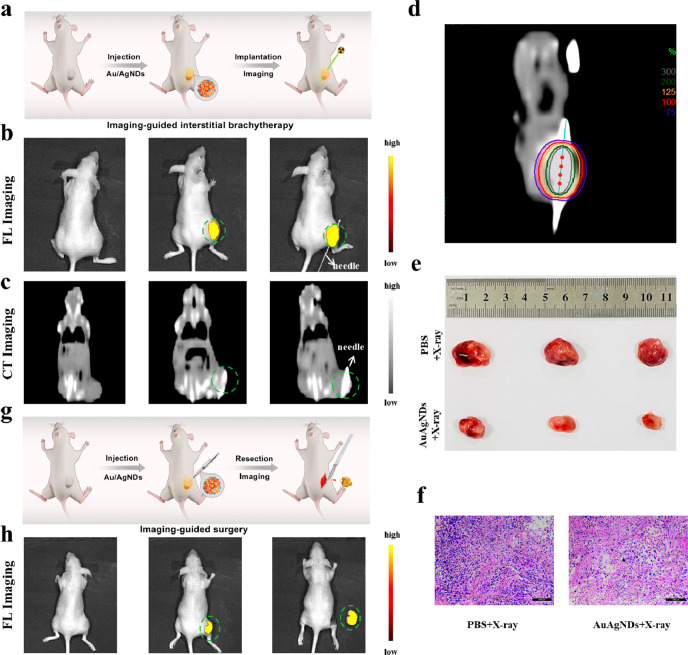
FL/CT dual-mode imaging guided interstitial
